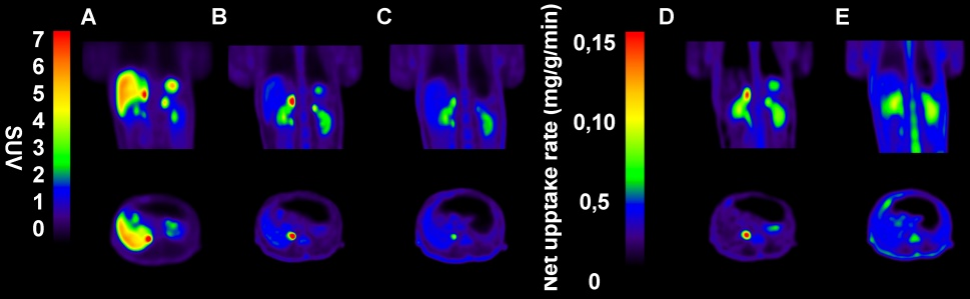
Representative sagittal (upper panel) and transverse (lower panel) PET images in NPH. A-C: SUV images 60-85 min post tracer injection. D-E: Net uptake rate (Patlak). A: [^11^C]MTO, B: [^18^F]CETO, C: [^18^F]CETO after blockage with etomidate 0.5 mg/kg, D: [^18^F]CETO, E: [^18^F]CETO after blockage with etomidate 0.5 mg/kg.
